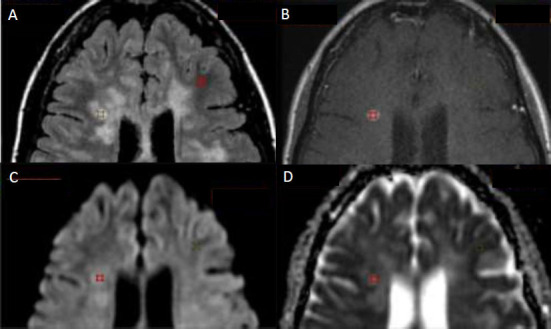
The locations of the region of interest (ROI) within the MS lesions, (A) Axial T2 fluid-attenuated inversion recovery (FLAIR) image, (B) axial SE T1 post-contrast image, (C) DWI image and (D) ADC image. The ROIs are located in the enhancing lesion and in the NAWM tissue.
